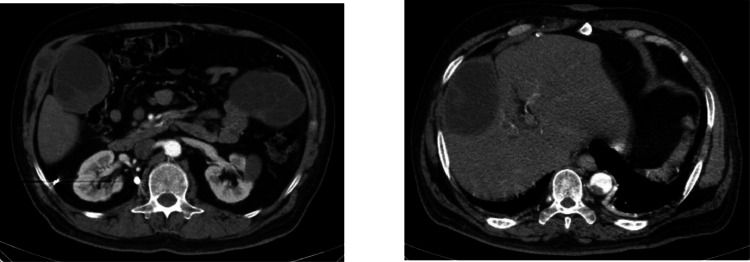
The CT revealed a normal-sized liver with a subcapsular, cystic structure in segment VIII measuring 98 × 65 mm, containing internal undulating membranes suggestive of a hydatid cyst. A similar cystic lesion measuring 93 × 61 mm was identified adjacent to segment VI, also consistent with hydatidosis. Further scrutiny identified a cystic structure in the left upper quadrant measuring 112 × 85 mm, with internal membranes and daughter cysts, suggestive of another hydatid cyst. There is evidence of a hyperdense suspiciously metallic foreign body adjacent to right side psoas muscle in perirenal space at L1 level.
